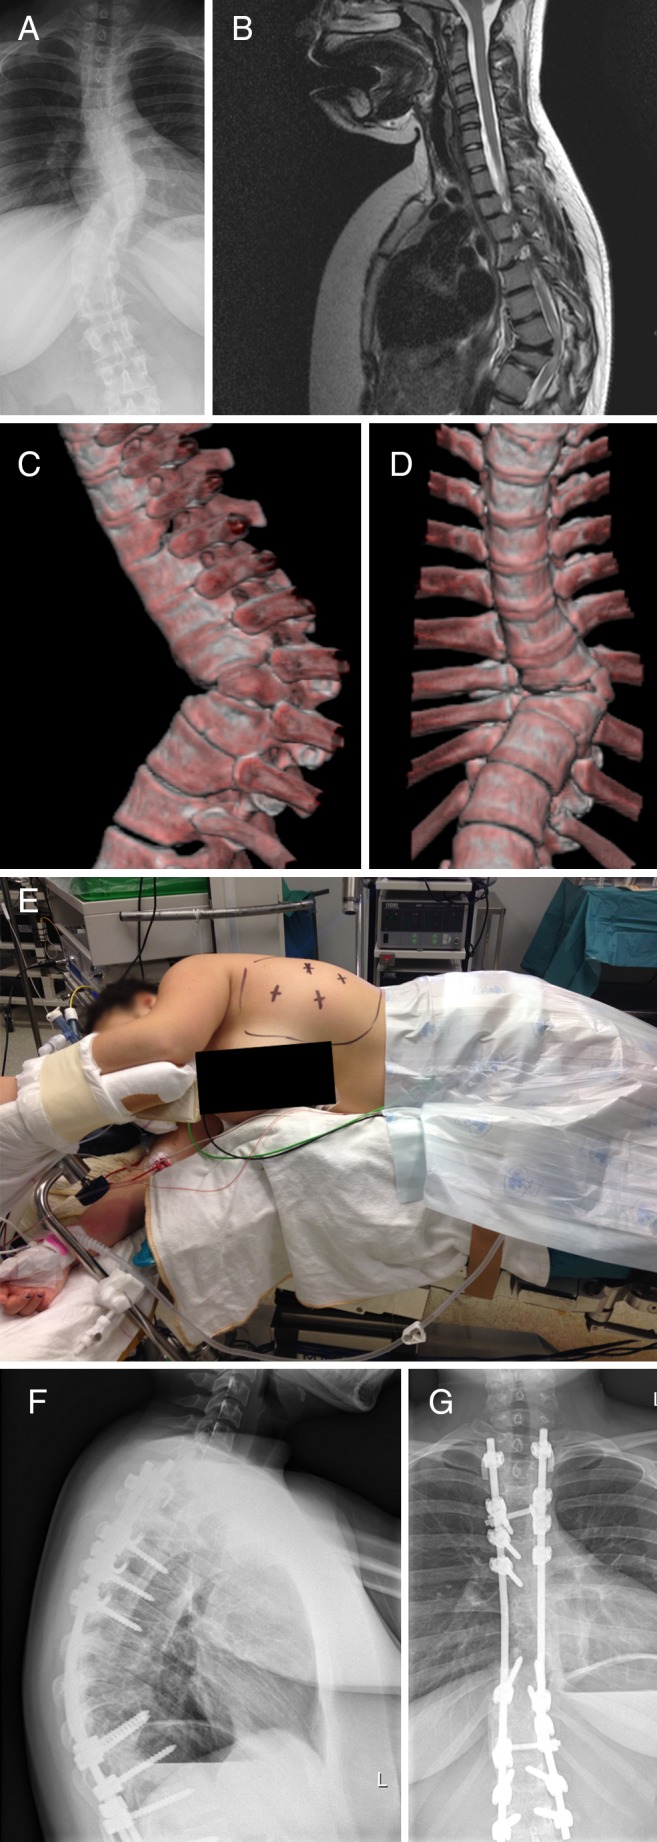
(Case 1): Girl with T8 hemivertebra, angular kyphosis, and slight scoliosis without any complaints. a Antero-posterior X-ray. b Sagittal T2-weighted MRI. c Lateral 3D CT reconstruction. d Antero-posterior 3D CT reconstruction. At age 15, the appearance of an intramedullary T2-hyperintens signal prompted prophylactic surgery, starting with an anterior thoracoscopic hemivertebrectomy and multi-level anterior release (e), followed by posterior redression and spondylodesis. Lateral (f) and antero-posterior (g) X-ray demonstrate partial correction of deformity
